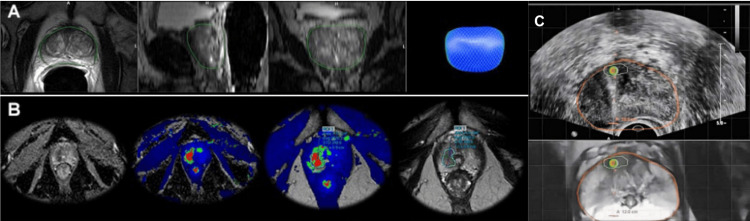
MRI/US-guided prostate biopsy. (A) MRI/US-guided biopsy of the prostate involves initially performing MRI scans of the prostate and rendering a 3D model of the prostate (blue spherical structure), including the location of the suspected tumour using these data. (B) Diffusion-weighted and ADC images can then further elucidate the position of the tumour (coloured green and red). (C) Subsequently, this 3D model, including the tumour’s position, is fused in real time with US imaging, allowing the operator to precisely sample the tumour. The orange line demarcates the outline of the prostate, and the suspected tumour outlined by a white line. Fusing the images allows the operator to accurately pinpoint the needle projection, as shown by the green and orange circles. ADC: apparent diffusion coefficient; MRI: magnetic resonance imaging; US: ultrasound.
